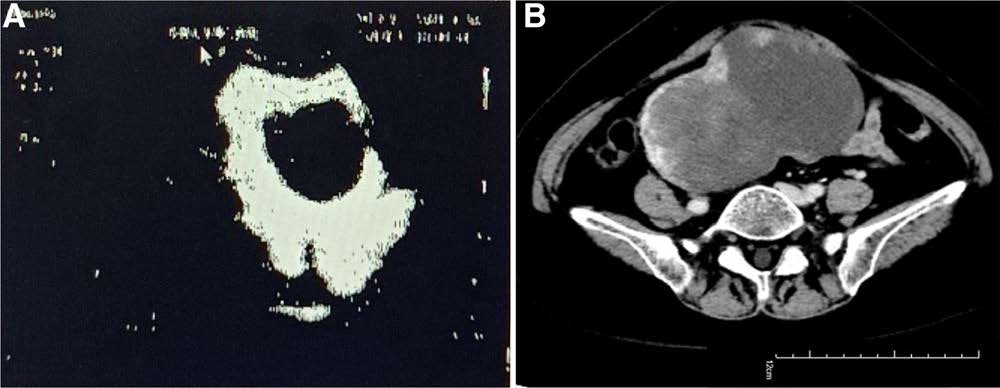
Abdominal ultrasonography (A) and computed tomography (B) presenting a large mass in the pelvis.
